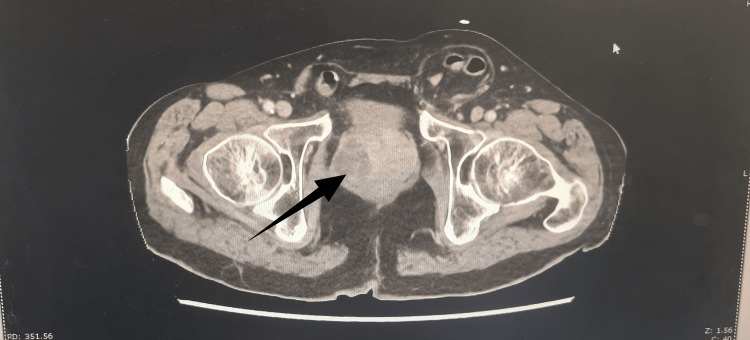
Contrast-enhanced CT scan of the pelvis in the axial view showing features suggestive of a prostrate abscess in the right lobe. The black arrow points to the abscess in the right lobe of the prostate.
